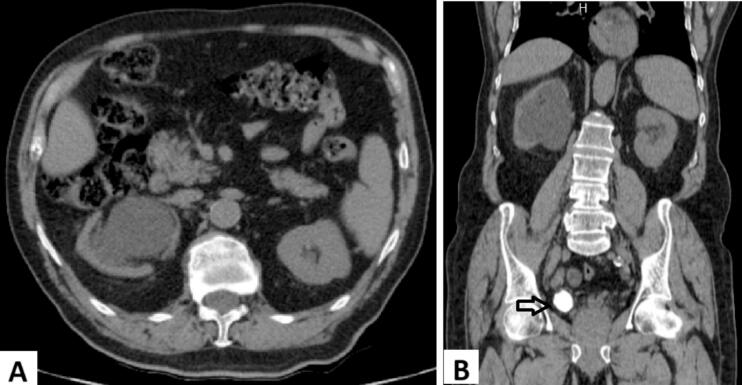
Preoperative computed tomography (CT) scan demonstrating severe right sided hydronephrosis with thinning of the right renal cortex. (A) Axial CT view, (B) coronal CT view. Arrow in (B) pointing to the large right distal ureteral stone.
